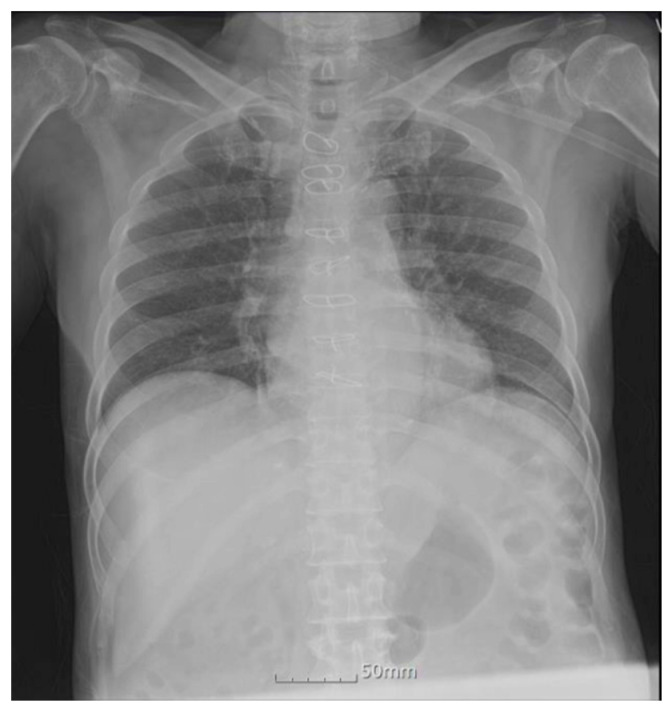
Chest radiography showed non-specific infiltrations at both lower lungs.
